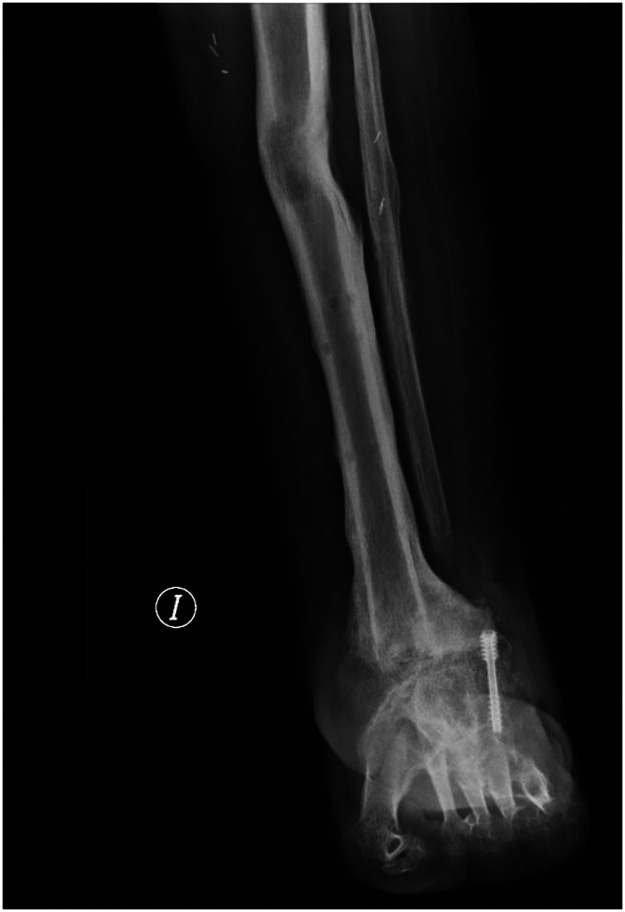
Patient's final radiographic status, displaying a residual deformity resulting from the pressure exerted by the pseudoaneurysm.
